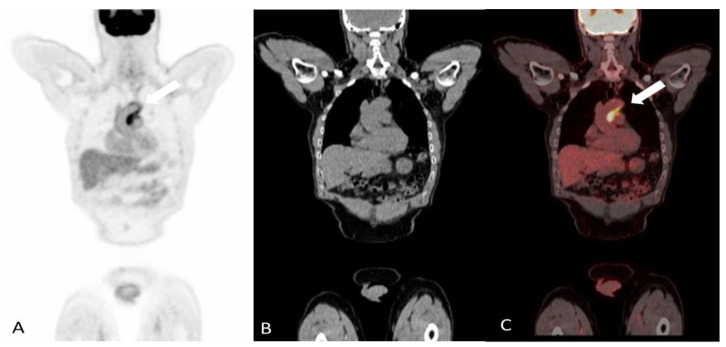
A 60-year-old woman with giant cell arteritis. Coronal images of attenuation corrected F-18 FDG PET (A), CT without contrast (B) and fused PET/CT (C) demonstrating moderate intensity linear increased tracer activity in the wall of the ascending aorta and proximal arch (arrows), most consistent with active vasculitis.
